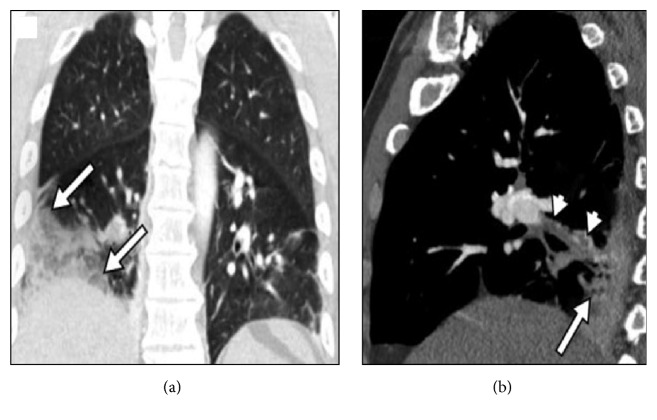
(a) Coronal CTA image in lung window shows infiltrate in the right lung base, suggestive of a peripherally located infarcted lung (arrows). (b) Sagittal CTA image shows embolism within the right lower lobe segmental branches (arrowheads) and the peripherally located infarcted lung (arrow) [10].
